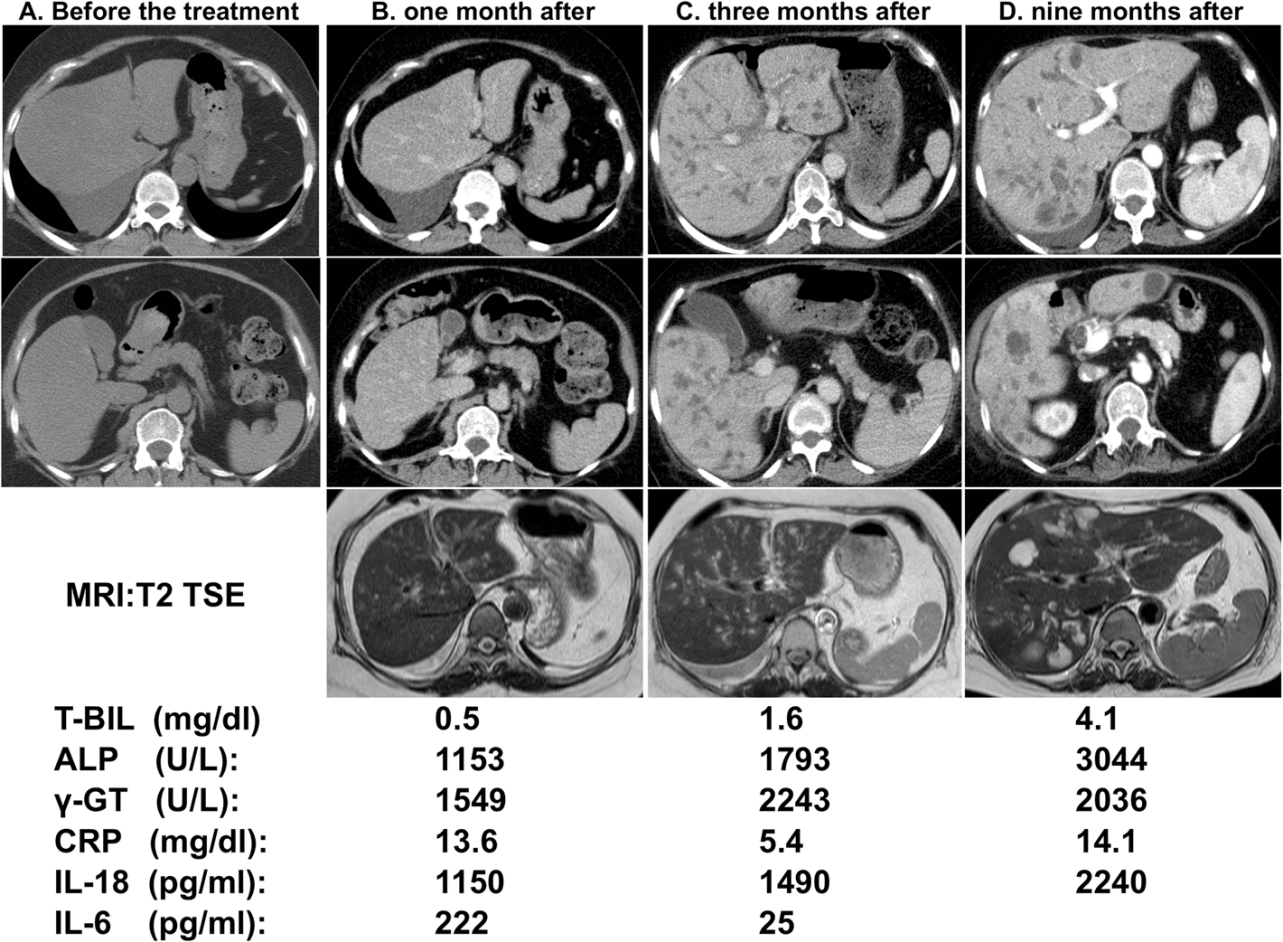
Computer tomography (CT), magnetic resonance imaging (MRI, T2 TSE) and laboratory results during the clinical course. a-d Abdominal CT and MRI before a, one month b, three months (c) and nine months (d) after treatment with ceritinib. a, b Abdominal CT and MRI showed no abnormalities of the liver. c CT showed a dilation of the intrahepatic bile duct and MRI also showed dilation of the intrahepatic bile duct with periportal edema. d CT and MRI showed a dilation of the intrahepatic bile duct and newly detected biloma. The results of serum T-Biil, ALP, γGT, CRP, IL-18 and IL-6 in the clinical course are shown under the imaging study. Serum IL-6 was decreased, but serum IL-18 was increased with the progression of the ceritinib-induced DIC even after its discontinuation and treatment with PSL
